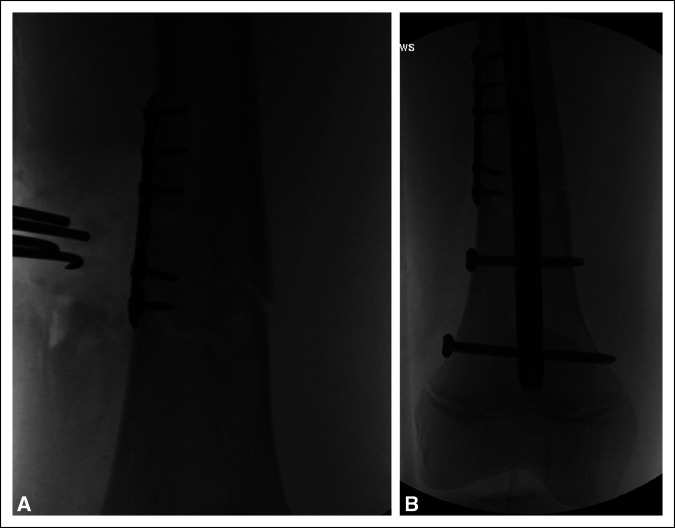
Radiographs showing A, anatomic reduction achieved with unicortical screws and plate and B, definitive fixation after a rigid intramedullary nail was inserted.
